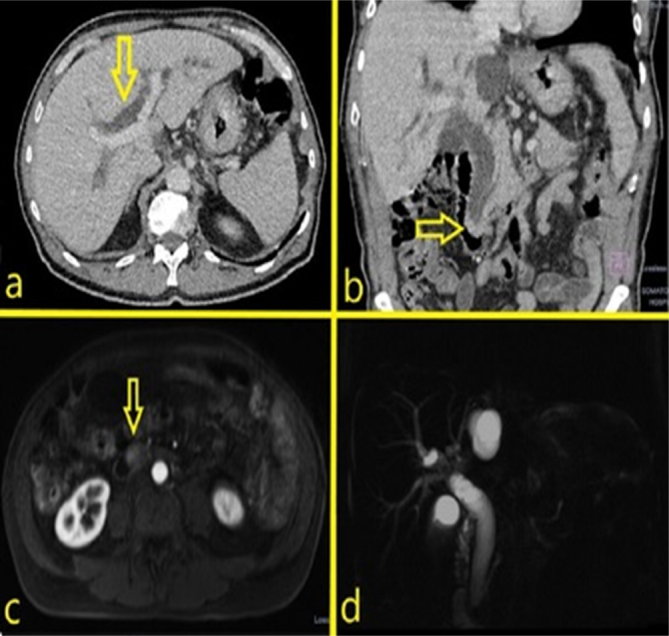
Preoperative upper abdominal computed tomography (a,b) and resonance
